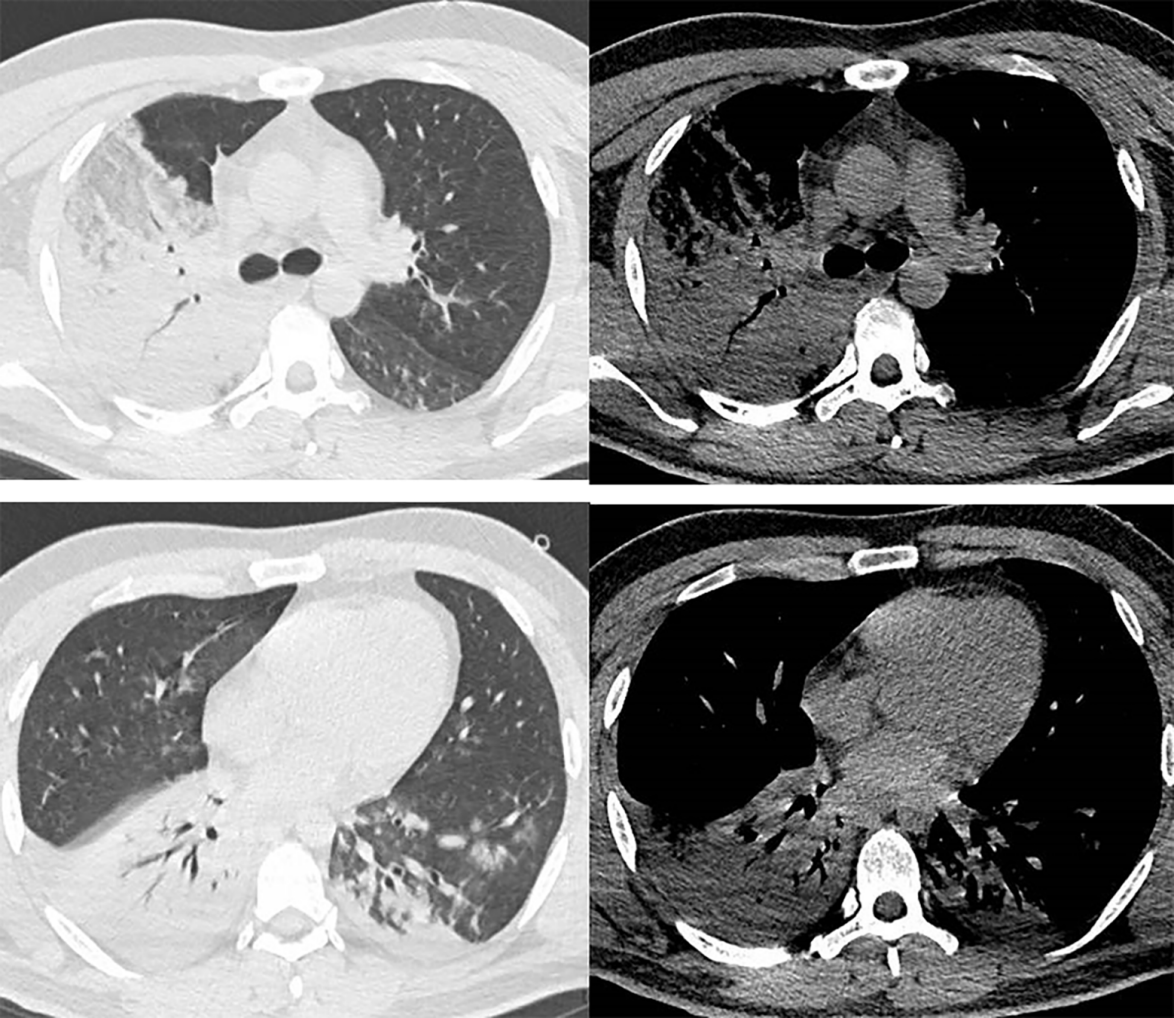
Images of chest computed tomography.
